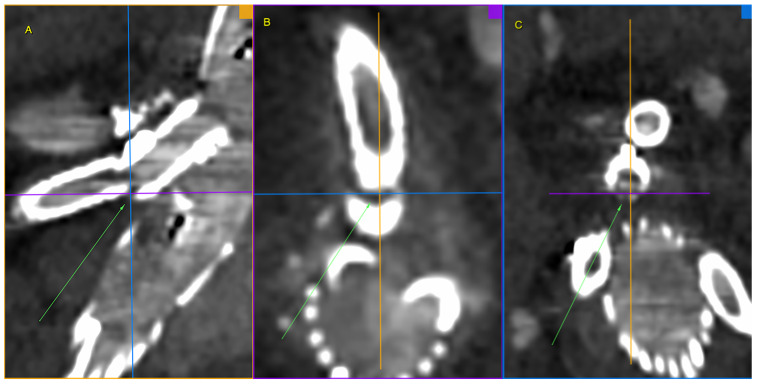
Ruptured superior mesenteric artery branch of stent graft in three planes. The fractured site is centrally located at the intersection of the lines defining the planes and additionally marked with green arrows. Panel (A)—sagittal projection, panel (B)—axial projection, panel (C)—coronal projection. Pre-interventional Angio-CT 3D MPR, arterial phase.
